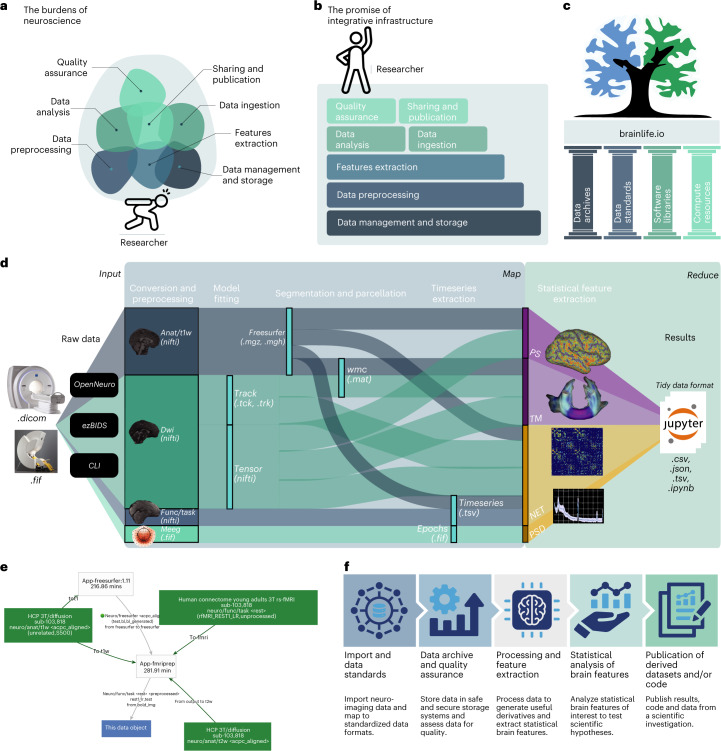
The burdens of neuroscience and the promise of integrative infrastructure. a, A figurative representation of the current major burdens of performing neuroimaging investigations. b, Our proposal for integrative infrastructure that coordinates services required to perform FAIR, reproducible, rigorous and transparent neuroimaging research thereby lifting the burden from the researcher. c, brainlife.io rests on the foundational pillars of the open science community such as data archives, standards, software libraries and compute resources. d, brainlife.io’s Map step takes MRI, MEG and EEG data and processes them to extract statistical features of interest. brainlife.io’s reduce step takes the extracted features and serves them to Jupyter Notebooks for statistical analysis. PS, parc-stats datatype; TM, tractmeasures datatype; NET, network datatype and CLI, common line interface. e, The brainlife.io technology automates capture of data provenance. All data objects on brainlife.io are stored with a record of the apps, app versions and parameters used to process the data. f, The primary services are provided to the user by brainlife.io. Panels a and b adapted from ref. ^22^ under a Creative Commons license CC BY 4.0.
